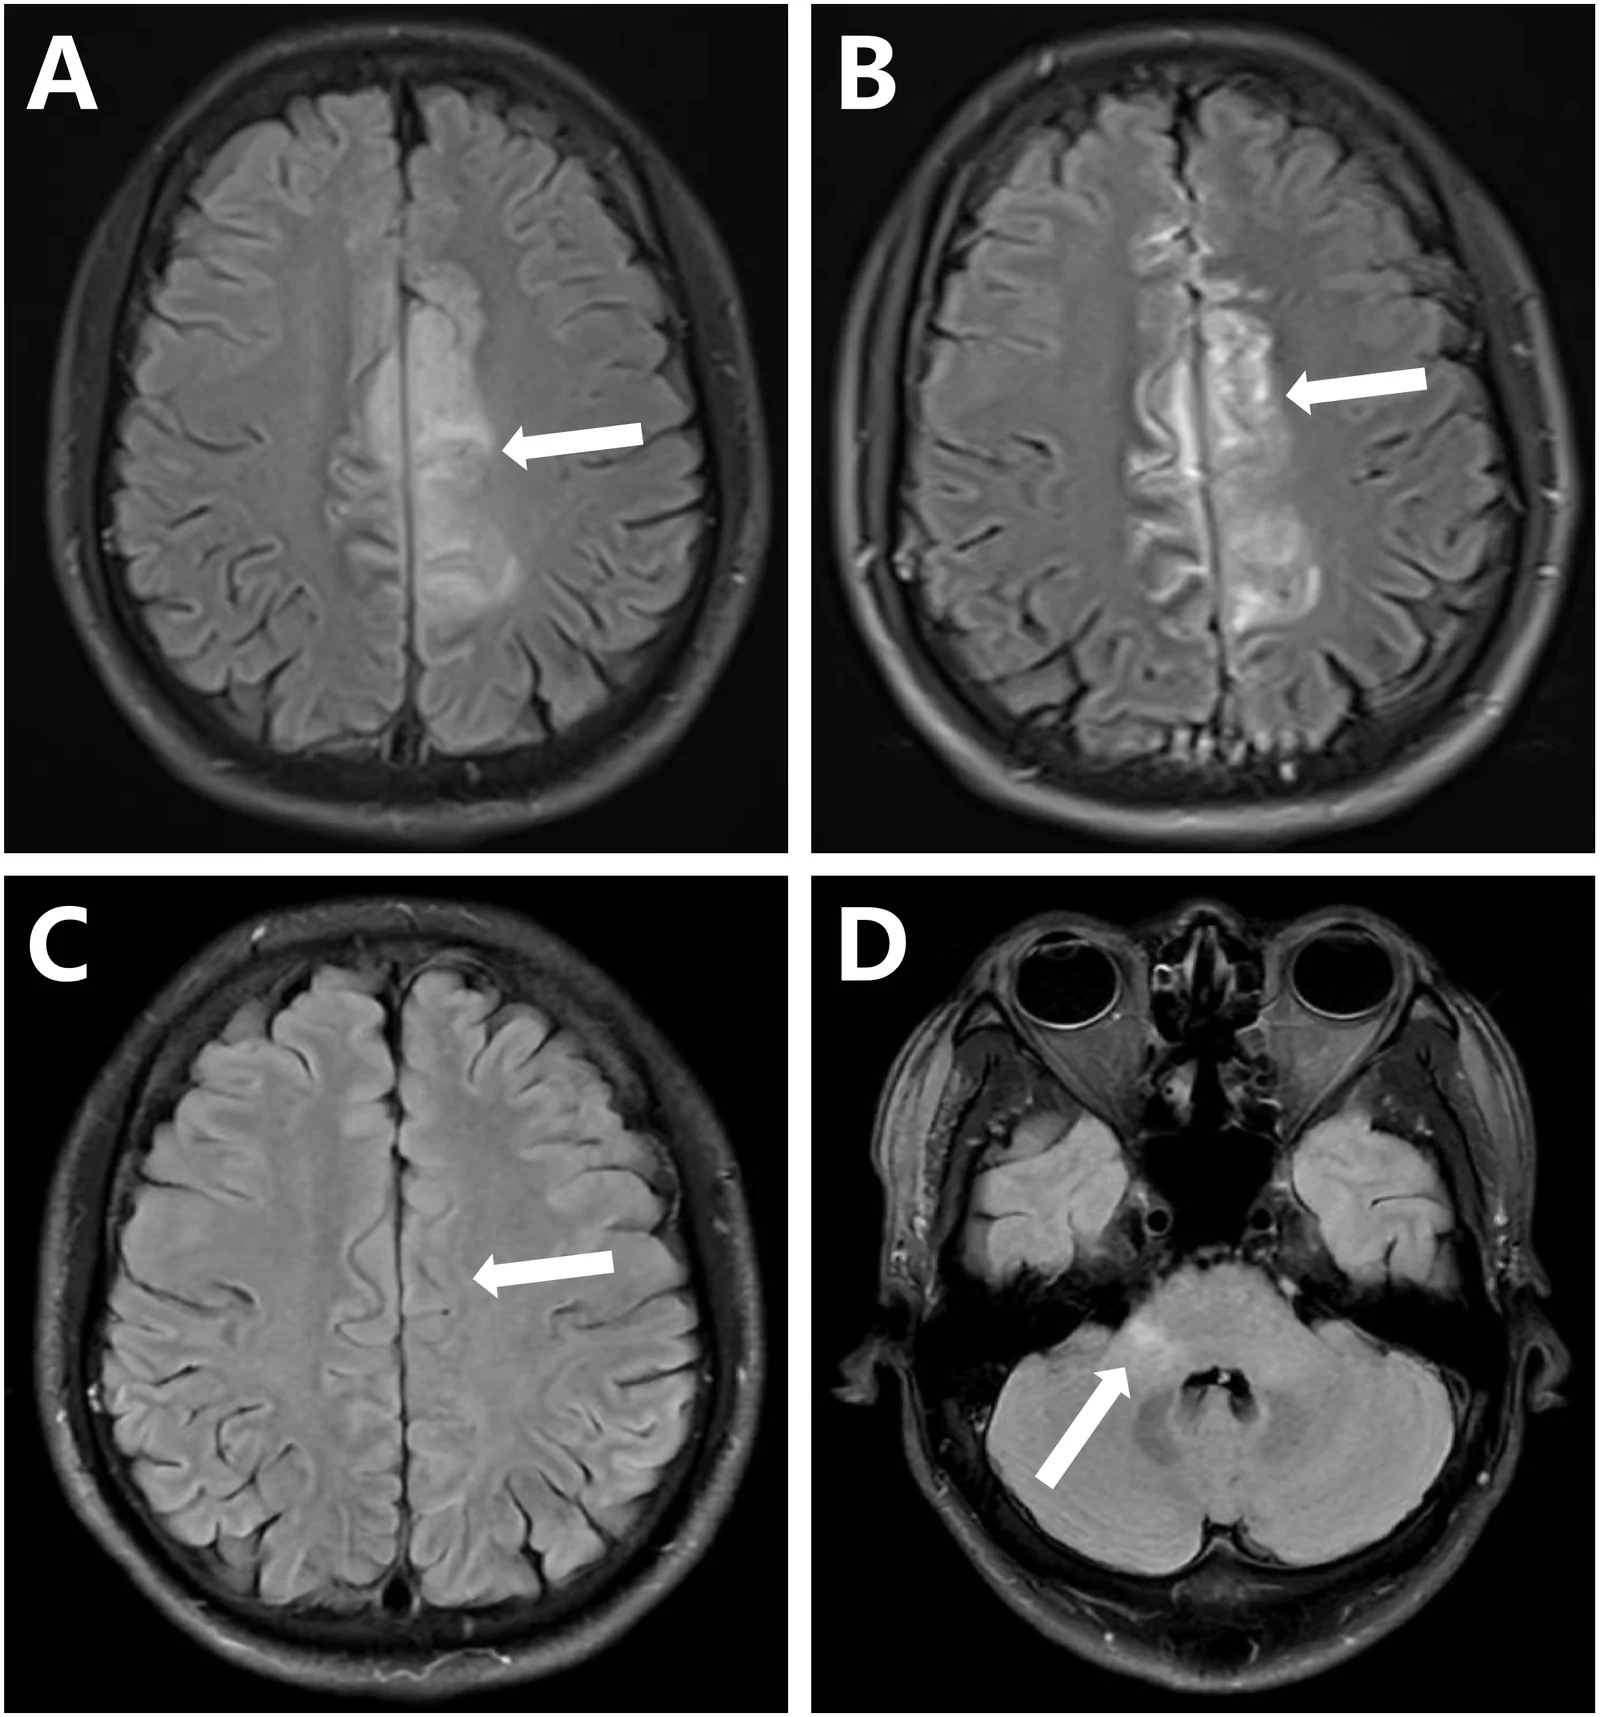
Brain MRI findings at initial onset and relapse in Case 1. (A, B) Brain MRI obtained at initial onset. (A) T2-FLAIR demonstrates hyperintense signal within the bilateral medial frontal cortex (white arrow). (B) Contrast-enhanced T2-FLAIR shows leptomeningeal enhancement over the bilateral medial frontal cortex (white arrow). (C) Follow-up T2-FLAIR at relapse demonstrates complete resolution of the previous bilateral medial frontal cortical lesions (white arrow). (D) New T2-FLAIR hyperintense lesion is observed in the right middle cerebellar peduncle at relapse (white arrow).
